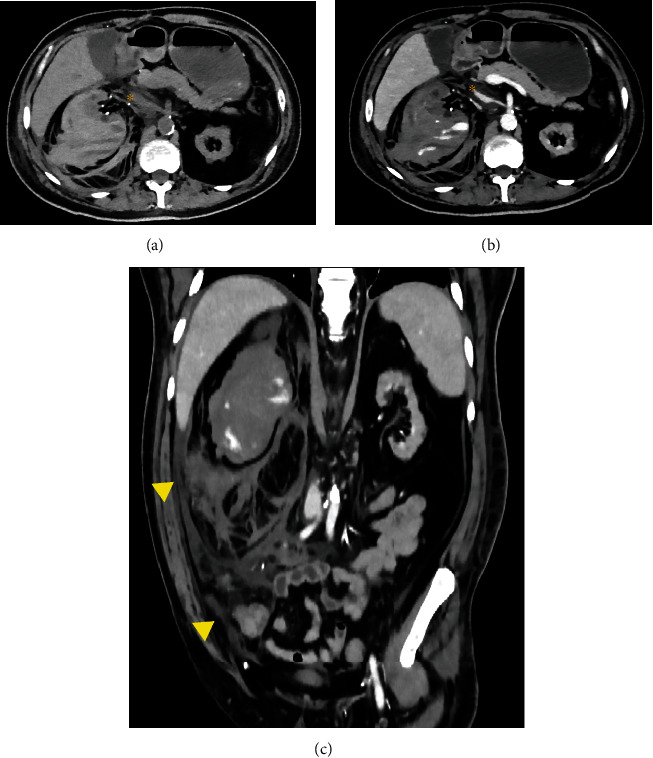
Axial nonenhancement (a) and venous phase CT (b) show increased right kidney size secondary to subcapsular and perirenal hematoma conditioning inferior vena cava displacement (^∗^). Coronal venous phase CT (c) shows hematic fluid along the right lateral paracolic gutter to the ipsilateral iliac fossa (arrowhead).
